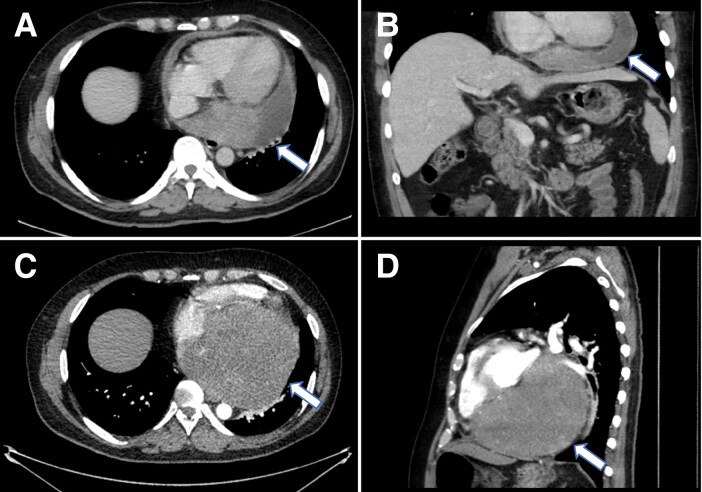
(*A*, *B*) Contrast computed tomography scan (using Omnipaque 350 contrast agent) showing a moderate-sized pericardial effusion predominantly accumulated in the posterior pericardial cavity, with a maximum depth of 4.5 cm. (*C*, *D*) Repeat contrast computed tomography scan (using Omnipaque 350 contrast agent) showed a significantly increased lesion in the pericardium compressing the left atrium and ventricle.
